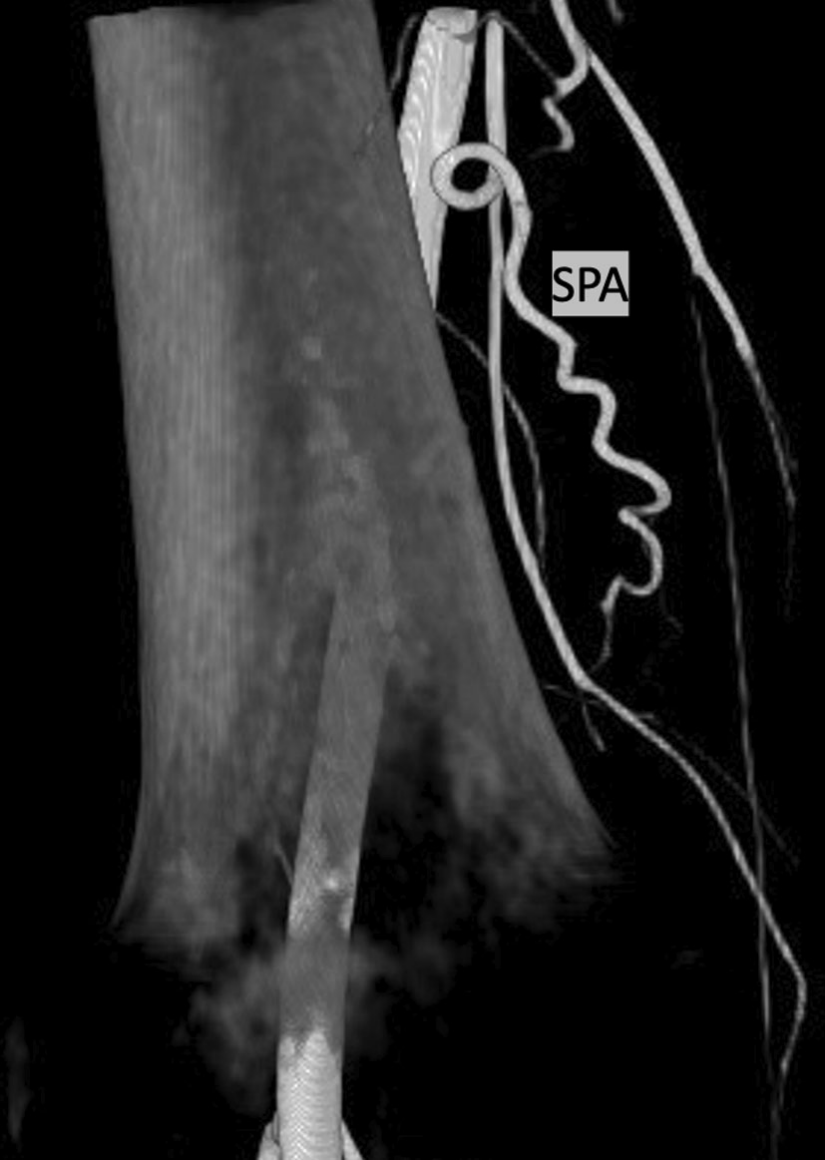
Intraoperative contrast-enhanced cone-beam computed tomography of the superior patellar artery
